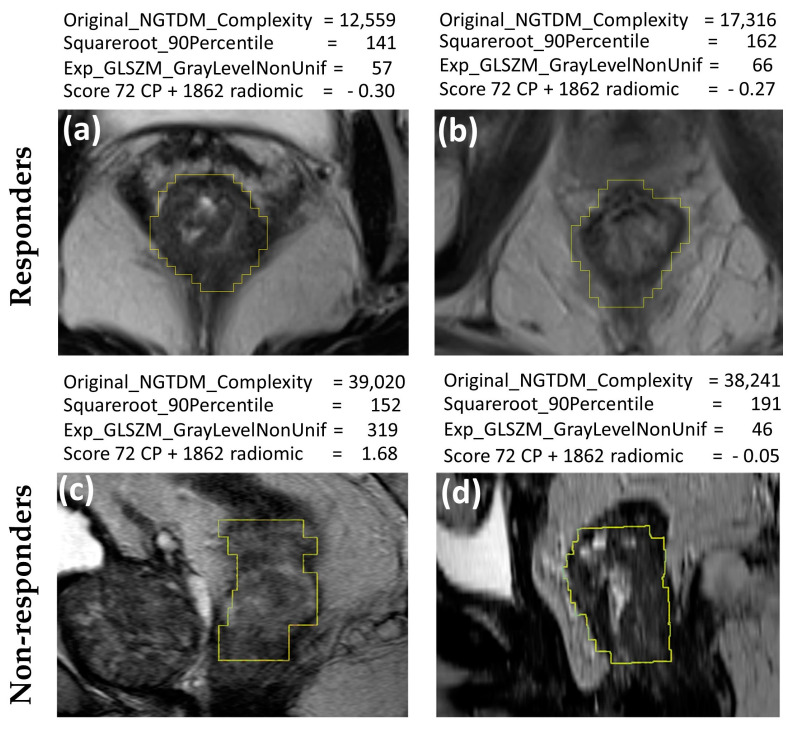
Examples of analyzed MRI images in the T2W contrast sequence sagittal plane (a,b) nCRT responders with cCR and (c,d) nCRT non-responders with TRG4. The values of several predictively prominent features selected for the predictive scores (Table 3) are indicated for each image. Notably, these feature values are in their native version, except for the score 72 CP + 1862 radiomics values, which have been z-score normalized.
